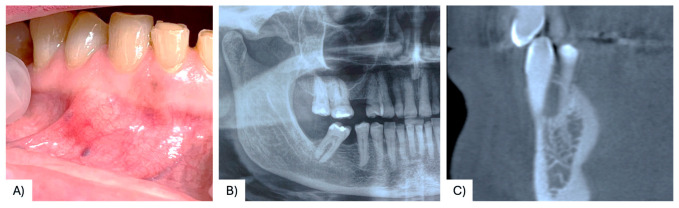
Small swelling of the vestibular gingiva in the region of #43 and #44 (A); radiograph showing a roundish radiolucency with radiolucent borders between elements #4.3 and #4.4 (B,C).
